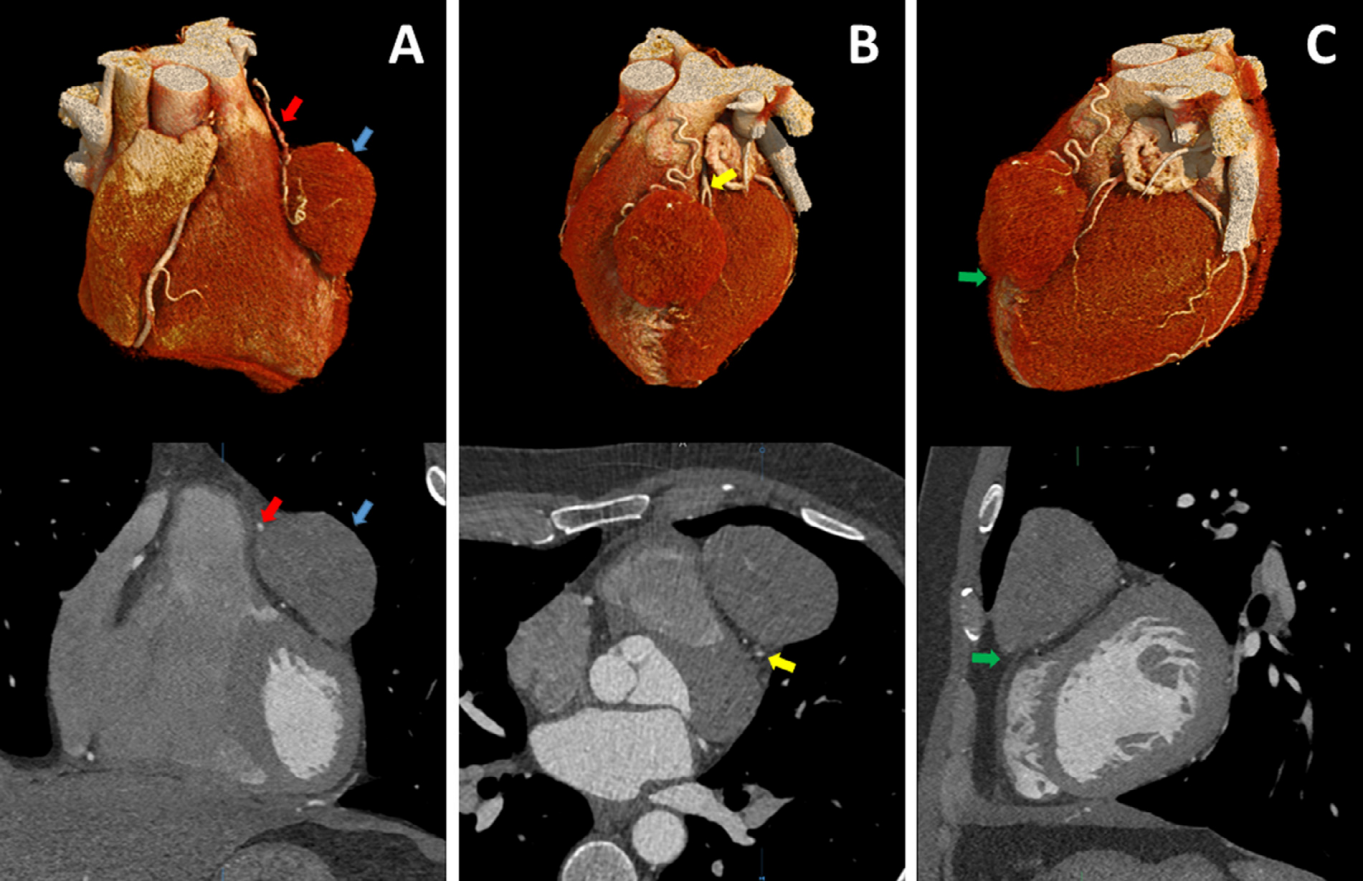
Cardiovascular computed tomographic angiography demonstrating mass borders (A, *blue arrows*), independent mass blood supply (A, *red arrows*), mass proximity to the left anterior descending coronary artery (B, *yellow arrows*), and left and right ventricles (C, *green arrows*).
